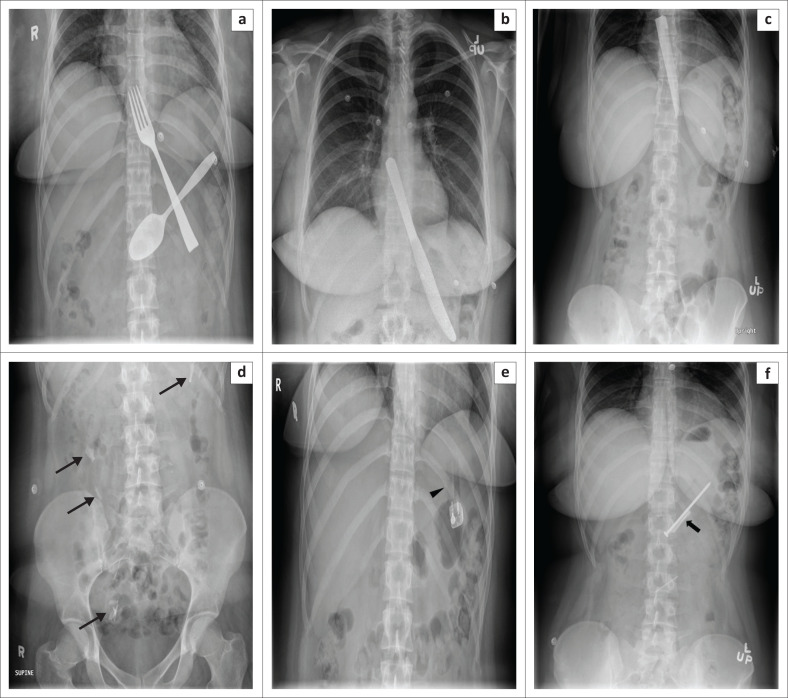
Serial chest and abdominal radiographs of a 25-year-old young woman taken over a period of one year with a history of physical and sexual abuse. The images demonstrate various intentionally ingested foreign bodies that included (a) a fork and spoon, (b) a table knife, and (c) a serrated knife, as well as various intentionally ingested foreign bodies that included (d) multiple glass pieces (thin arrows), (e) a cigarette lighter (arrowhead), and (f) a nail and a razor blade (thick arrow).
